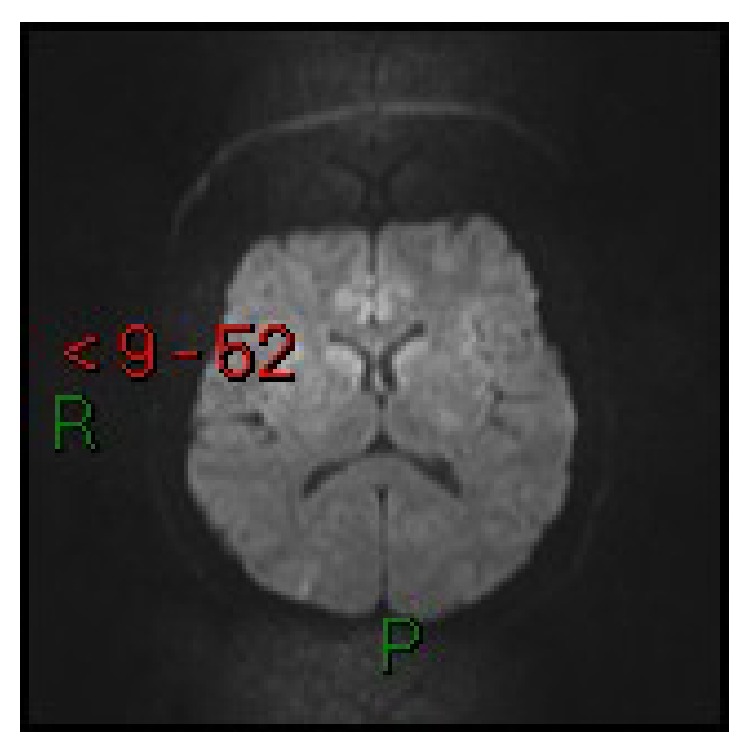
Case 1. Axial diffusion-weighted MRI: lesions do not present diffusion restriction.
